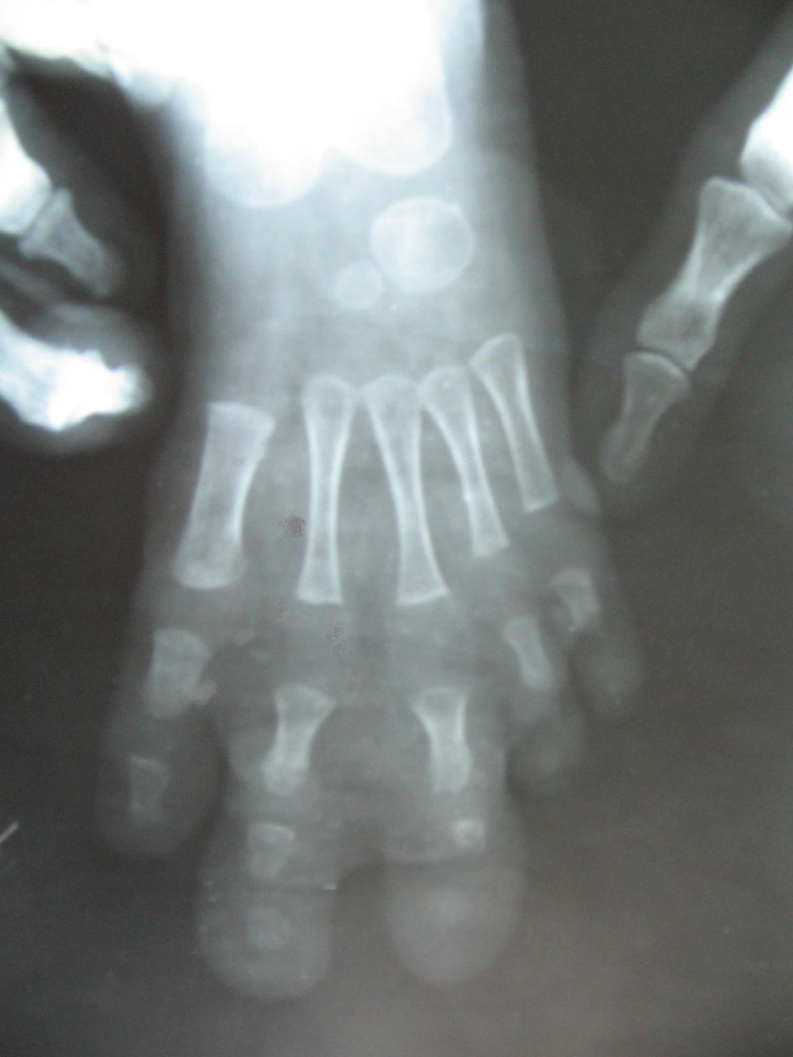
Enlarged 2^nd ^and 3^rd ^toes with well appreciated increase in size of metatarsals, phalanges and soft tissues on x-ray.
